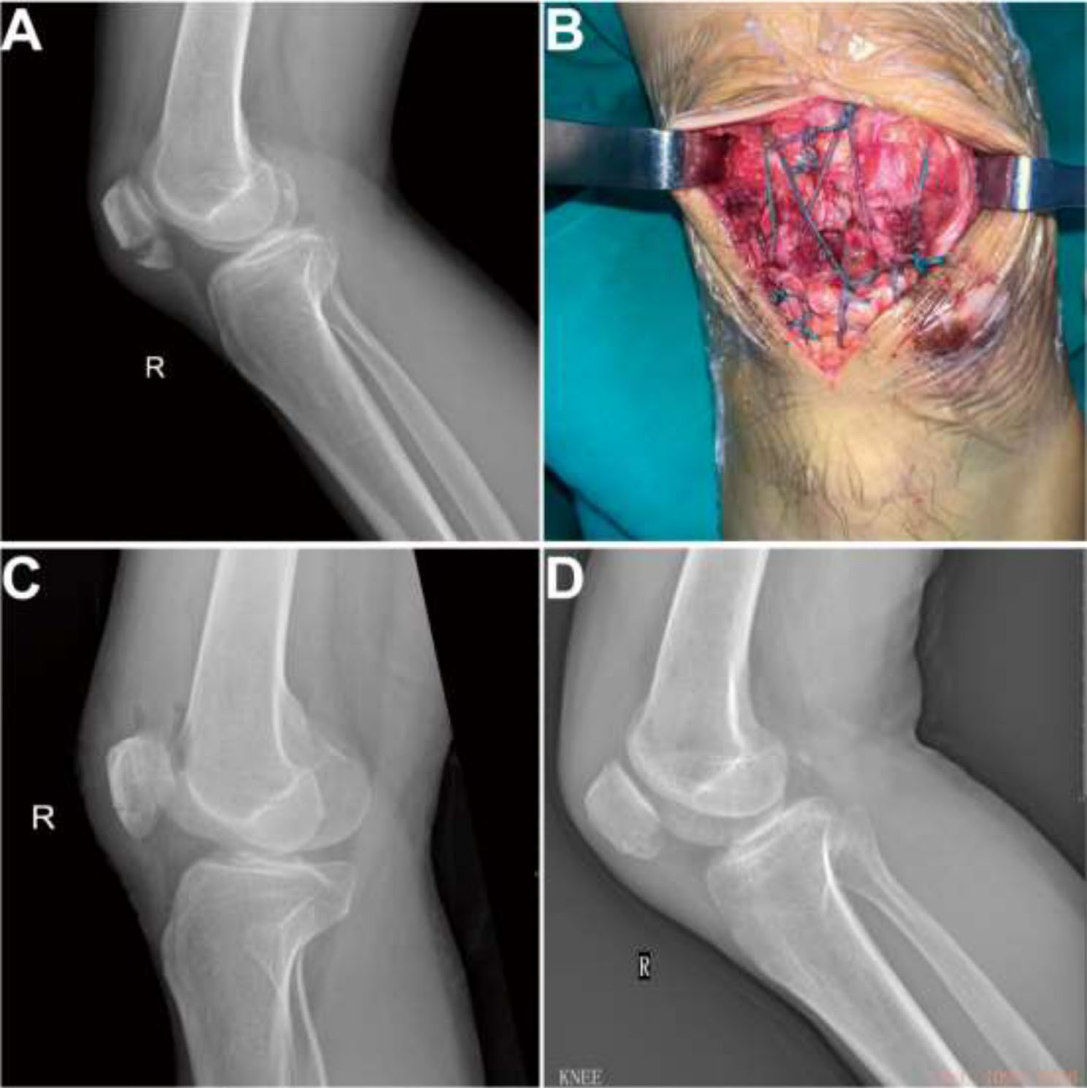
Case vignette No. 1. A 41-year-old man with a simple inferior pole patella fracture (right) from a fall injury with an AO classification of 34-A1 underwent Krackow suturing combined with the suture-bridge procedure. (A) Preoperative X-ray presentation; (B) Inferior pole patella fracture pulled by Krackow suturing and fixed by a suture bridge via turning over the free arms of the sutures; (C) Postoperative X-ray presentations; (D) X-ray presentations at 15 months after the operation
